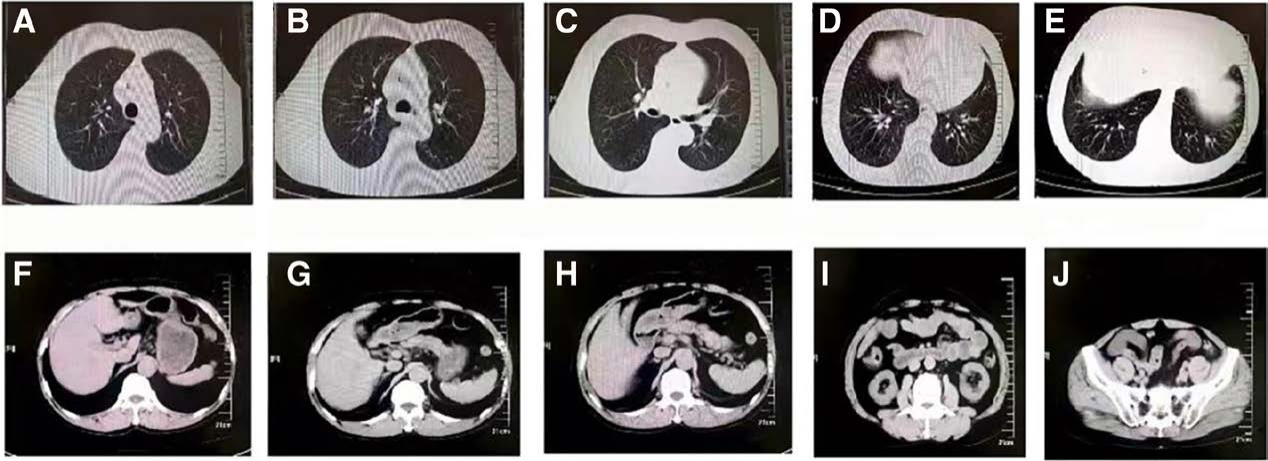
Changes in chest and abdominal computed tomography (CT) at the time of patient admission. (A–E) Changes in the thoracic CT at the time of patient admission. (F–J) Changes in abdominal CT of the patient upon admission. CT = computed tomography.
